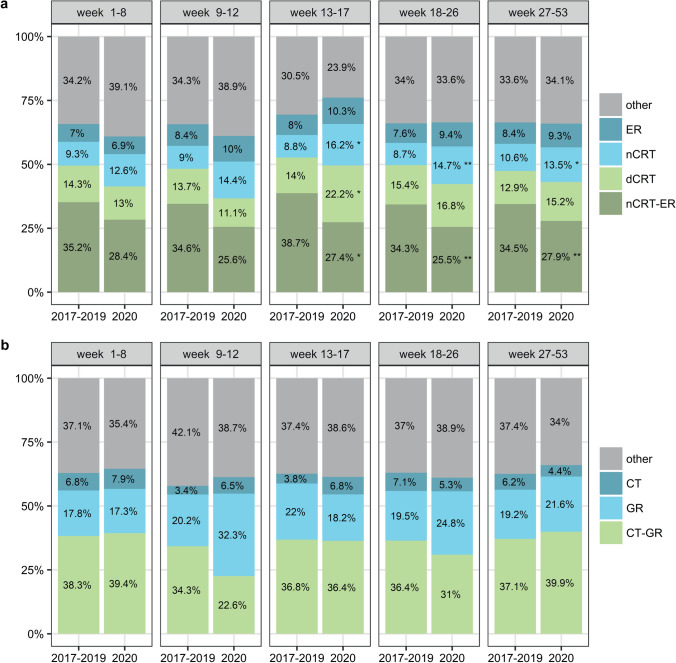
Plot of the overview of treatments given in the curable setting stratified by period of diagnosis where a shows the treatments of patients with potentially curable esophageal cancer and b shows the treatments in patients with potentially curable gastric cancer. nCRT-ER; neoadjuvant chemoradiotherapy with esophageal resection, dCRT; definitive chemoradiotherapy, nCRT; neoadjuvant chemoradiotherapy without resection, ER; esophageal resection without neoadjuvant chemoradiotherapy, CT-GR; gastric resection with (neo)adjuvant chemotherapy, GR; gastric resection without chemotherapy, CT; chemotherapy without resection
